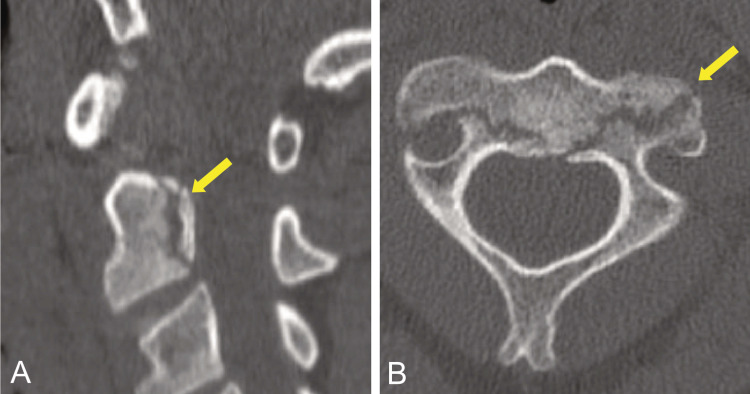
Computed tomography scans (A) Left paramidline (yellow arrow) and (B) fractures (yellow arrow) extending to the region of the foramina transversaria bilaterally.
